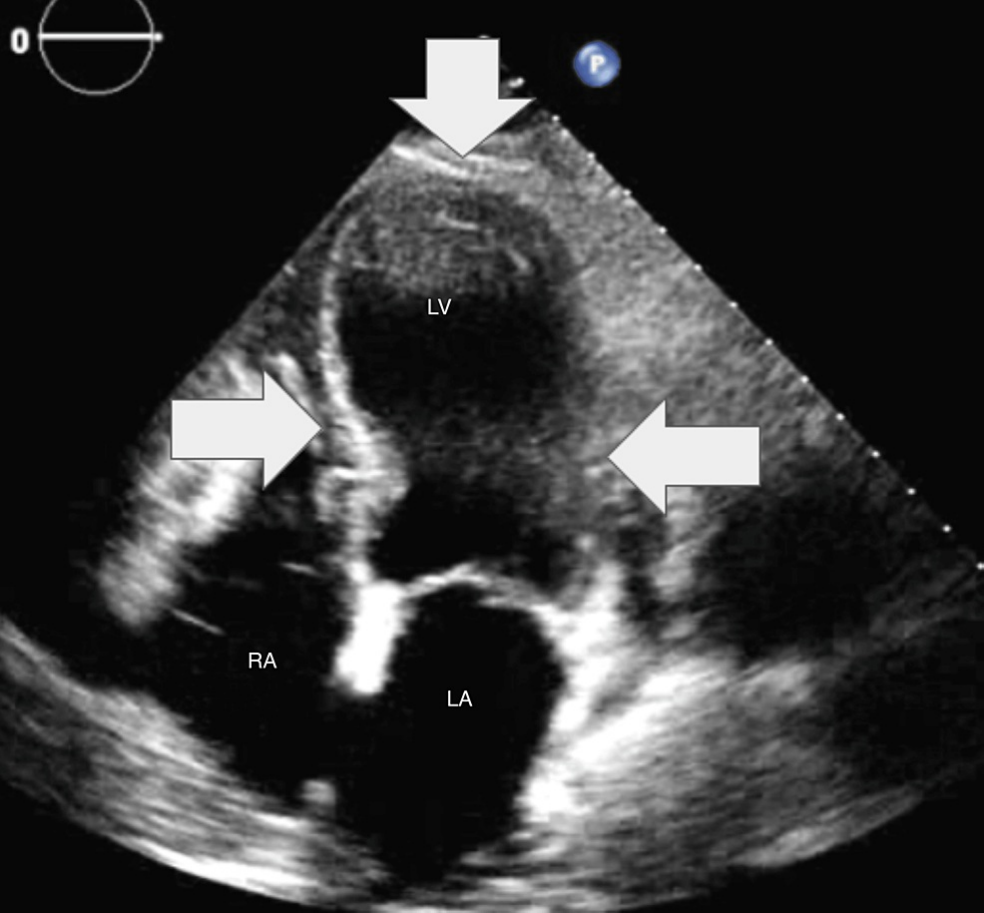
Apical four-chamber transthoracic echocardiogram (end-systolic frame) demonstrating apical ballooning with hypokinesis/akinesis and reduced ejection fraction LV: left ventricle, LA: left atrium, RA: right atrium
